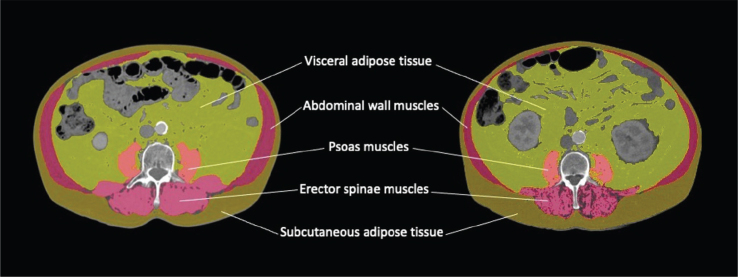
Body composition analyses were performed on axial computed tomography slices at the level of the 3rd lumbar vertebra. The male patient on the left had a normal skeletal muscle index (SMI) value of 55.4 cm^2^/m^2^ (normal ≥ 48.4 cm^2^/m^2^ for males). The male patient on the right had a low SMI value of 41.S cm^2^/m^2^.
